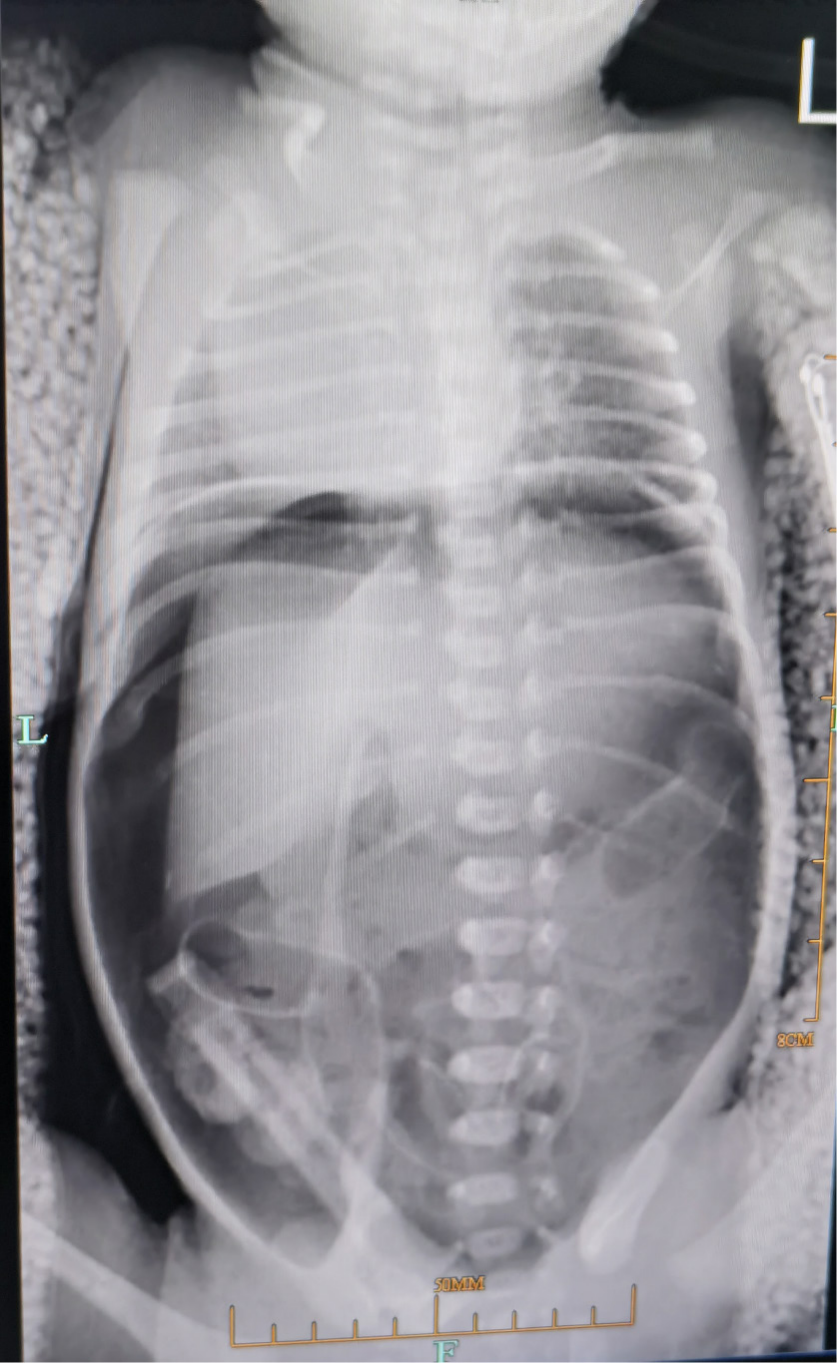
Abdominal radiograph of the female patient in the upright position.
